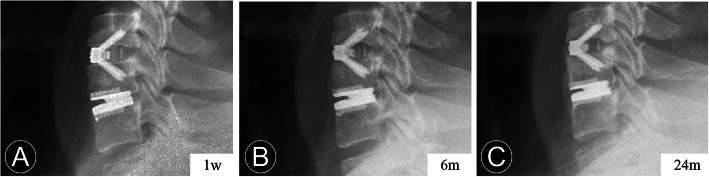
Serial postoperative radiographs of a 40-year-old man who underwent contiguous 2-level hybrid surgery at C5-C7. a and b The immediate and 6-month postoperative lateral radiographs show the peri-prosthesis bone loss and anterior heterotopic ossification. c At the last follow-up, the lateral radiograph shows the anterior heterotopic ossification developed
